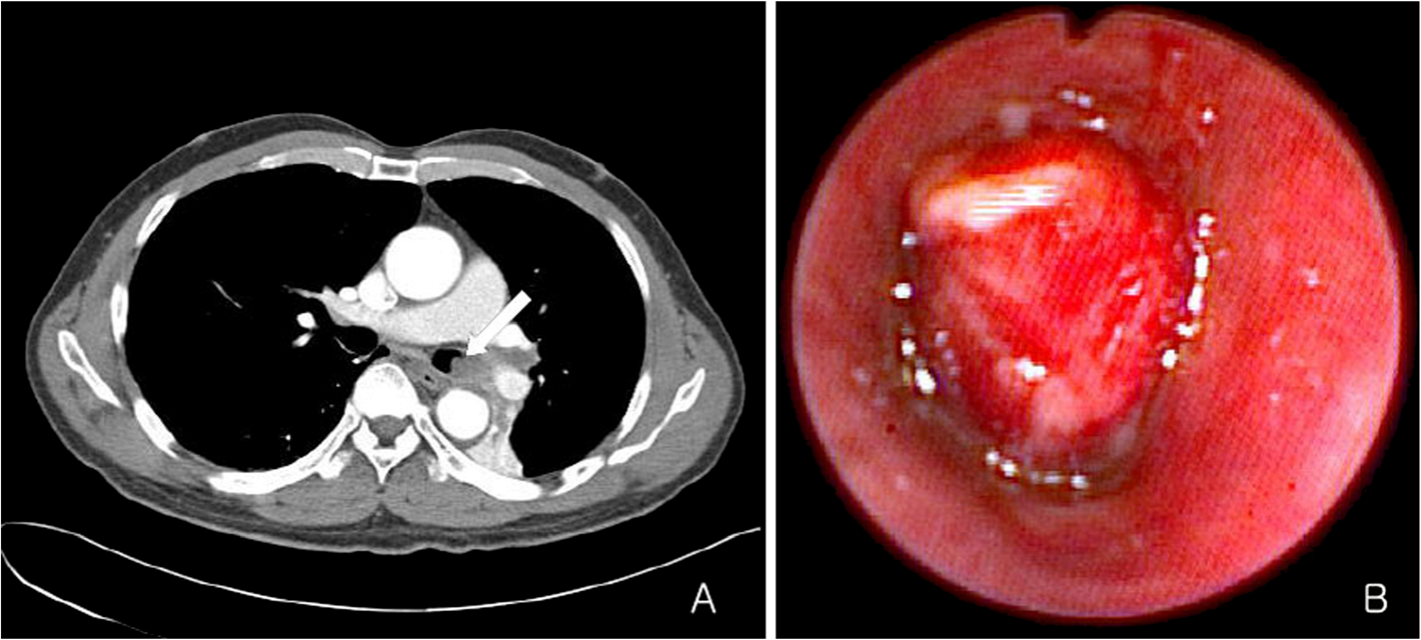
An endobronchial tumor (arrow) in the distal left main bronchus was shown in the chest computed tomography (A) and bronchoscopy (B).
